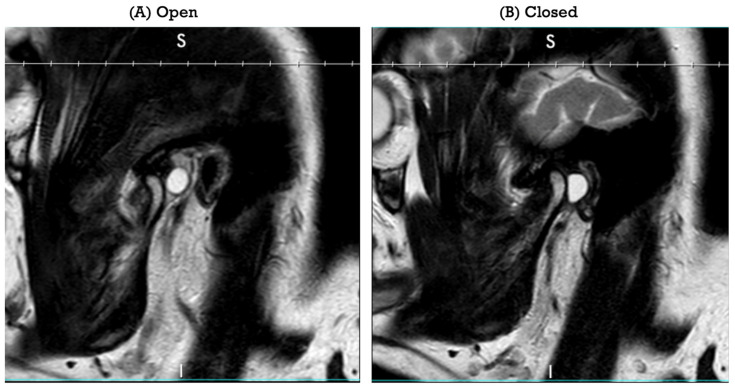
Sagittal view magnetic resonance images of the left temporomandibular joint. (A) View with mouth open. (B) View with mouth closed, demonstrating the posterior cystic lesion and its relationship to the external auditory canal. MRI sagittal views obtained with the mouth open (left) and closed (right).
